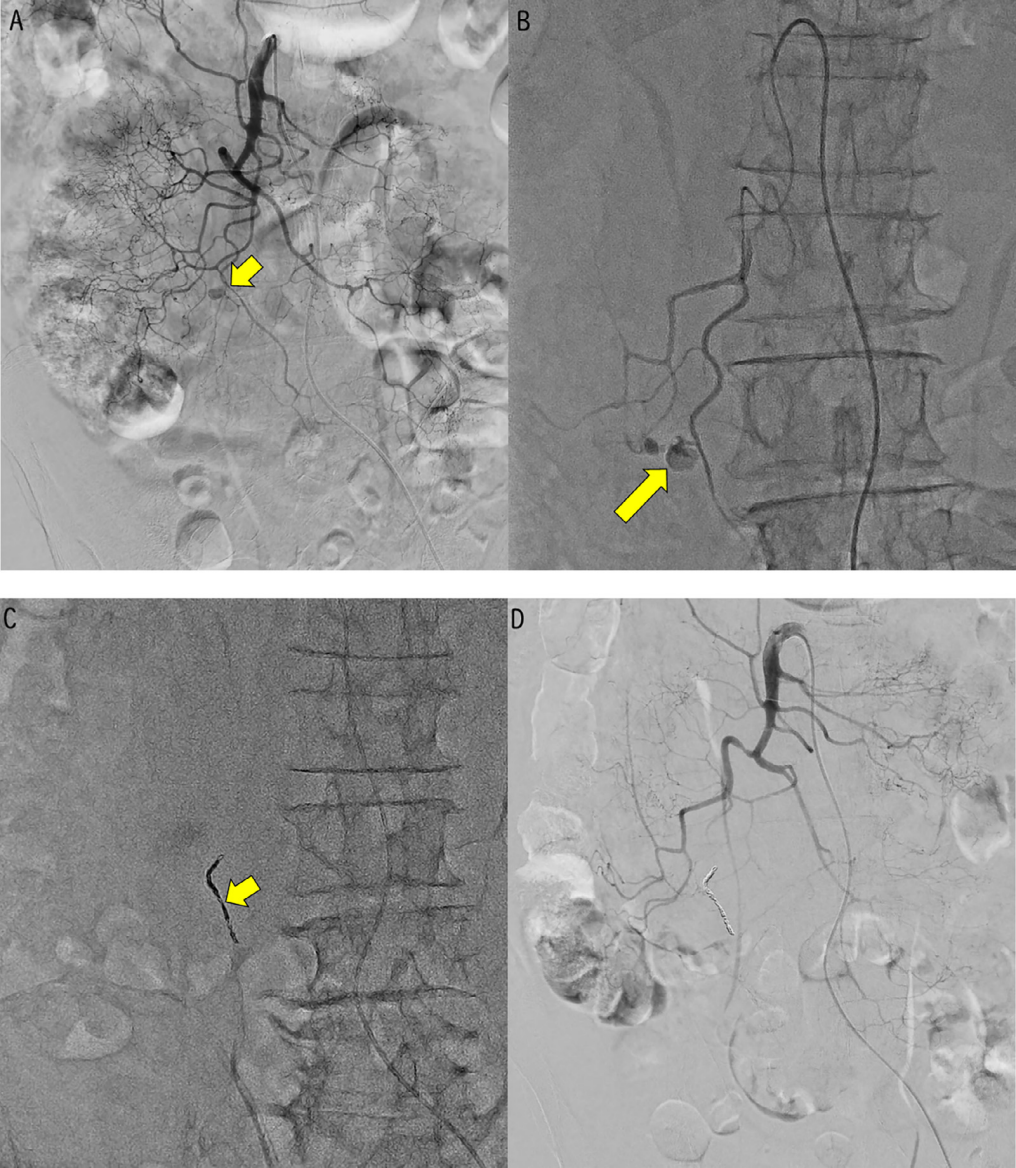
Angiography. (A) Selective digital subtraction angiography (DSA) of the superior mesenteric artery (SMA). (B) Selective digital angiography of the ileocolic artery. (C) Coil status after embolization on X-ray. (D) Postembolization DSA. Selective angiography of the SMA revealed extravasation from a branch of the ileocolic artery (A, B, arrow). Coil embolization was performed using detachable microcoils (C, arrow). Postembolization DSA confirmed successful hemostasis at the site of injury, with no evidence of active extravasation. Collateral blood flow to the terminal ileum and ascending colon was preserved via the marginal arteries (D).
